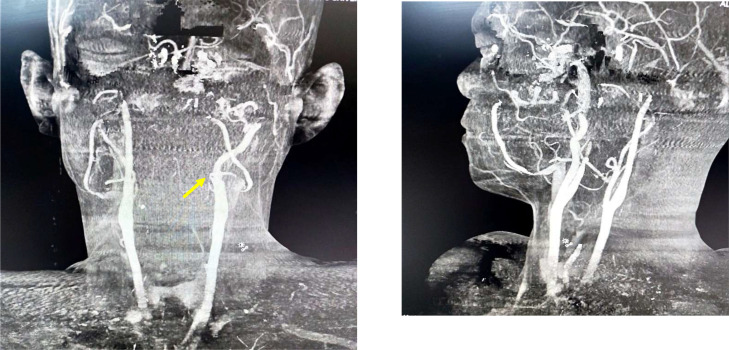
CT angiography, 3D vascular mapping of the head and neck. The yellow arrow highlights the atypical direct arterial supply originating from the left external carotid artery.
